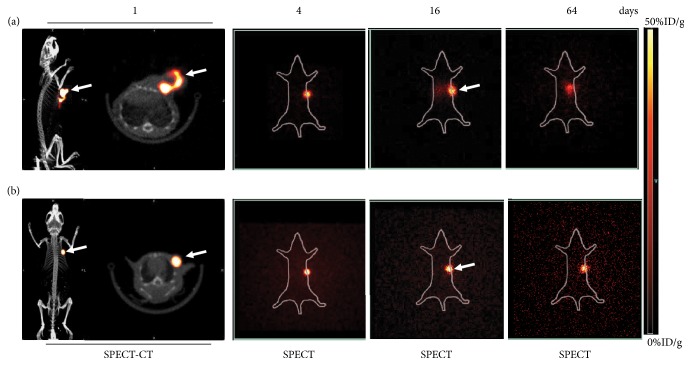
Representative micro-SPECT/CT or micro-SPECT images in the SG and CG. Micro-SPECT/CT images on day 1 and micro-SPECT images on days 4, 16, and 64 were obtained after intratumoural injection of colloidal ^32^P-CP ((a) ^32^P-CP colloids were in irregular shapes with leak from injection site and much notable abnormal distribution and liver appearance) and intratumour implantation of ^32^P-CP-PLGA seeds ((b) ^32^P-CP-PLGA seeds demonstrated the shape of a dot. No significant difference or appearance of other organs was observed until 64 days). The white arrows refer to tumors.
